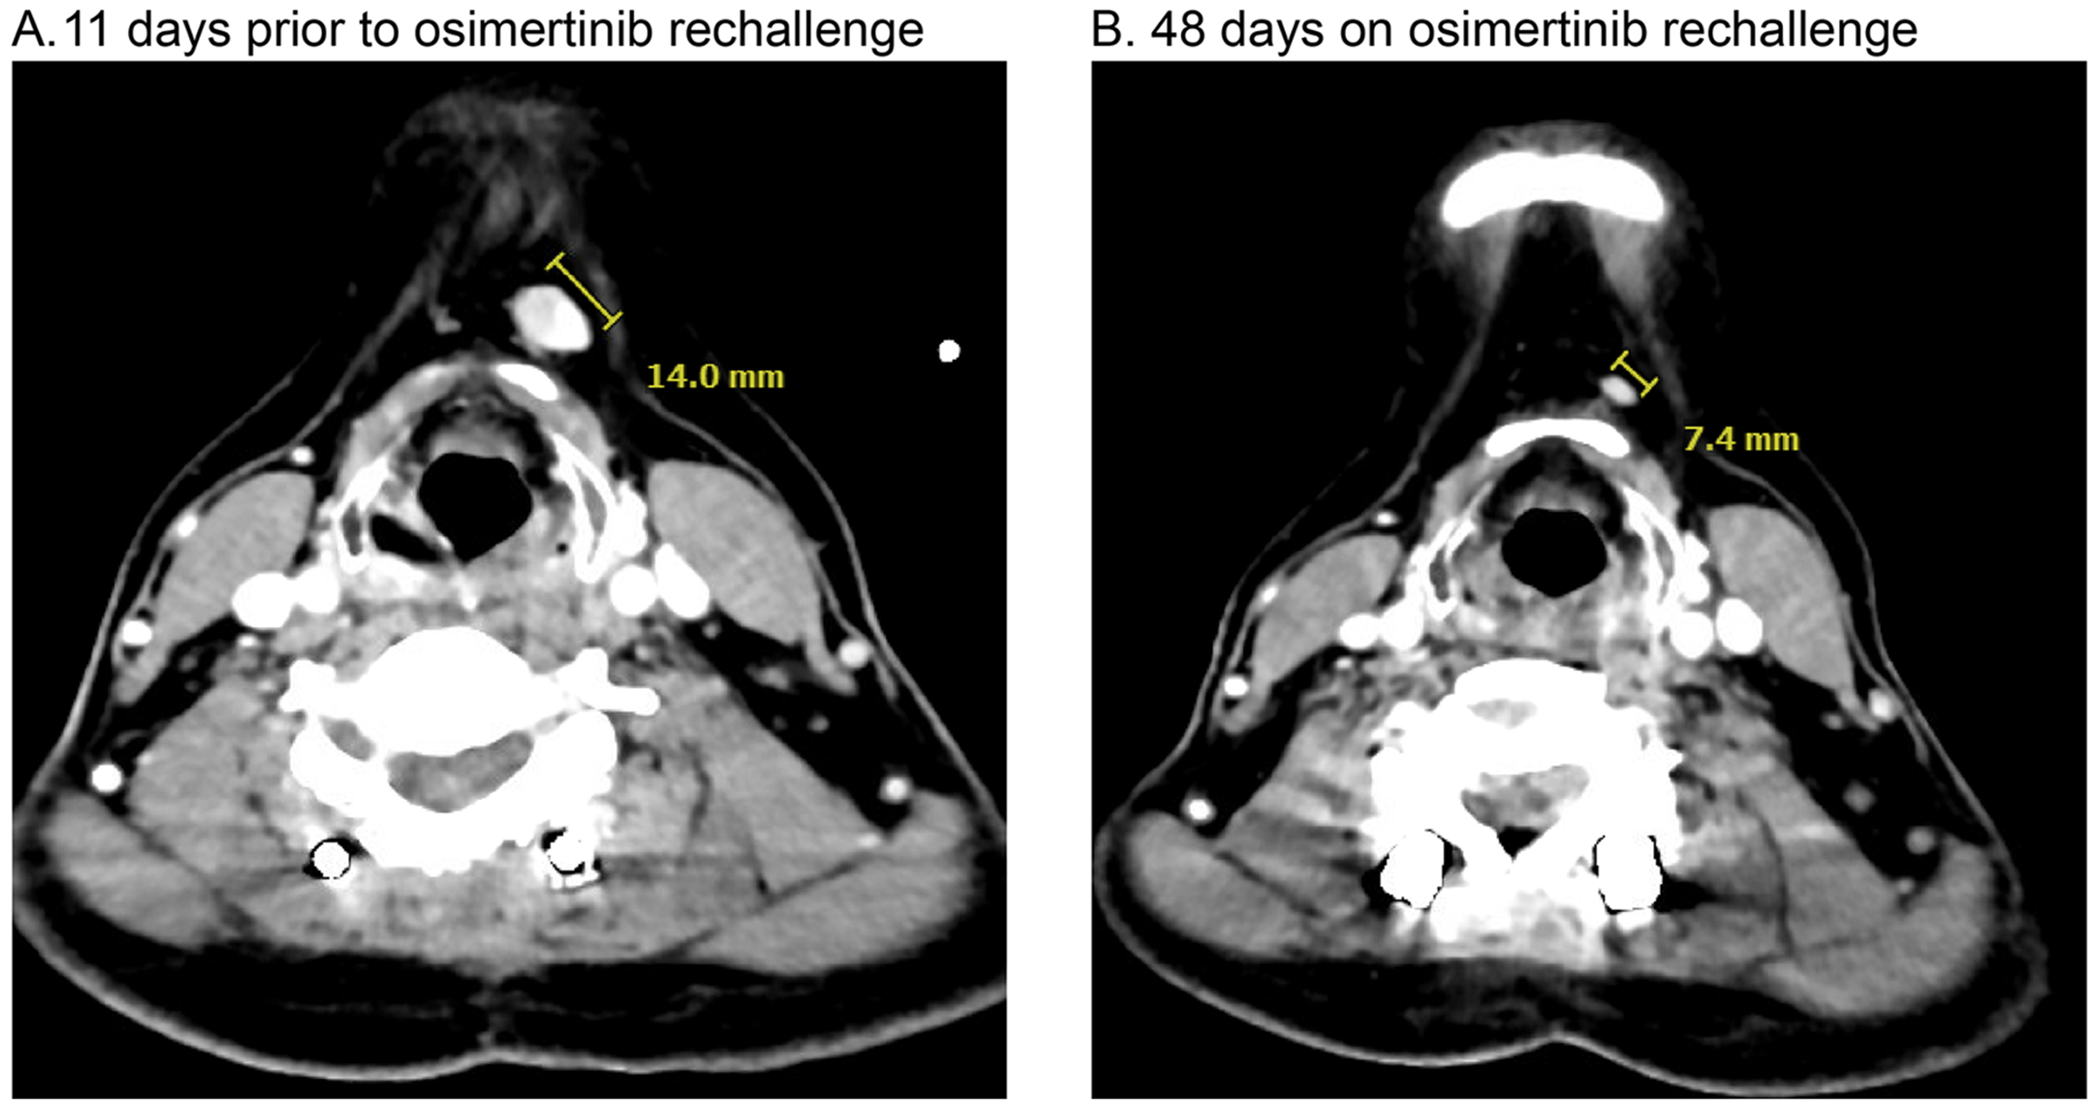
Radiographic response for patient 5 for osimertinib rechallenge. A) CT Neck w/ contrast image shows a 14 mm left level 1A submental lymph node 11 days prior to osimertinib rechallenge. B) CT Neck w/ contrast image shows that this lymph node has decreased in size to 7 mm 48 days following re-initiation of osimertinib.
